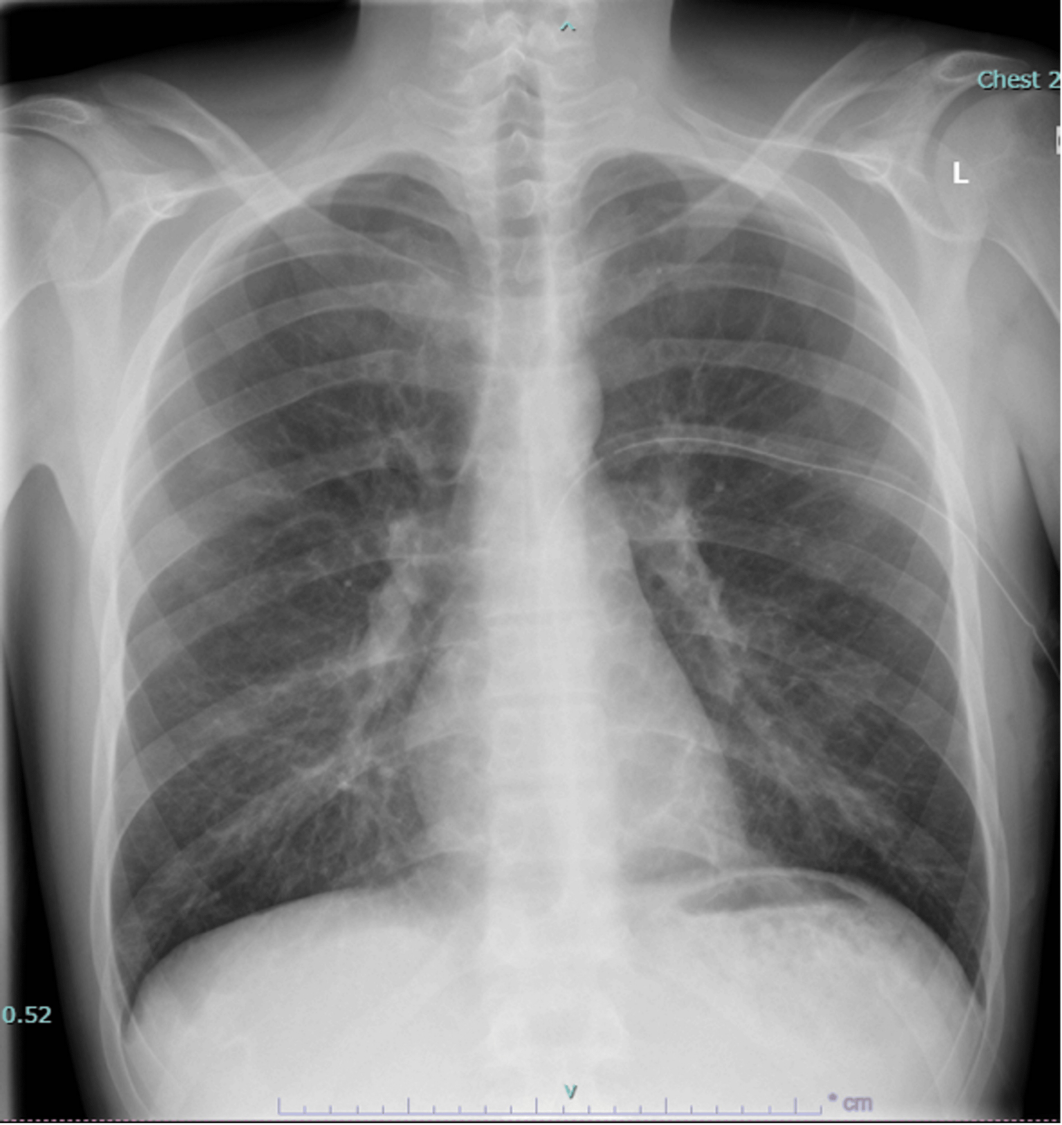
Normal chest X-ray with no acute cardiopulmonary process.
